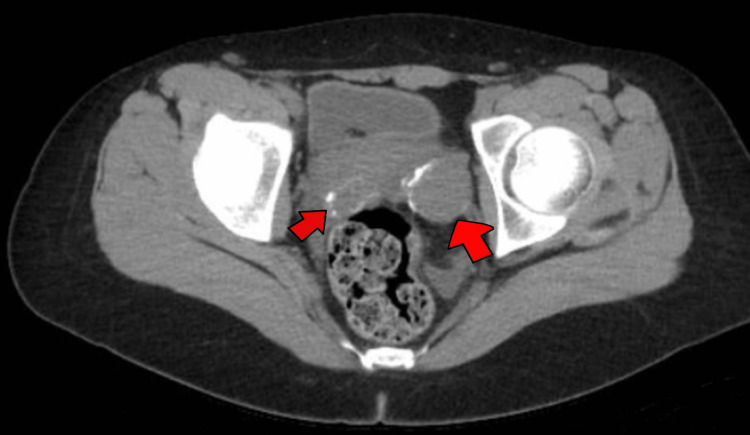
Computed tomography scan of the pelvis The exam revealed oval images with intermediate density and calcifications in bilateral adnexal region (arrows), measuring 30 mm on the right side and 28 mm on the left side. The findings were suggestive of primary gonadal tumoral lesions.
